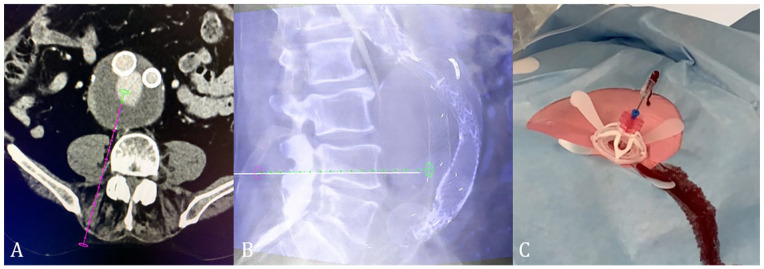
The needle puncture route (A) on preoperative computed tomography angiography (CTA), with needle guidance software based on intraoperative cone-beam computed tomography (CT) (B), and return of blood from the needle, indicating puncture of endoleak cavity (C).
